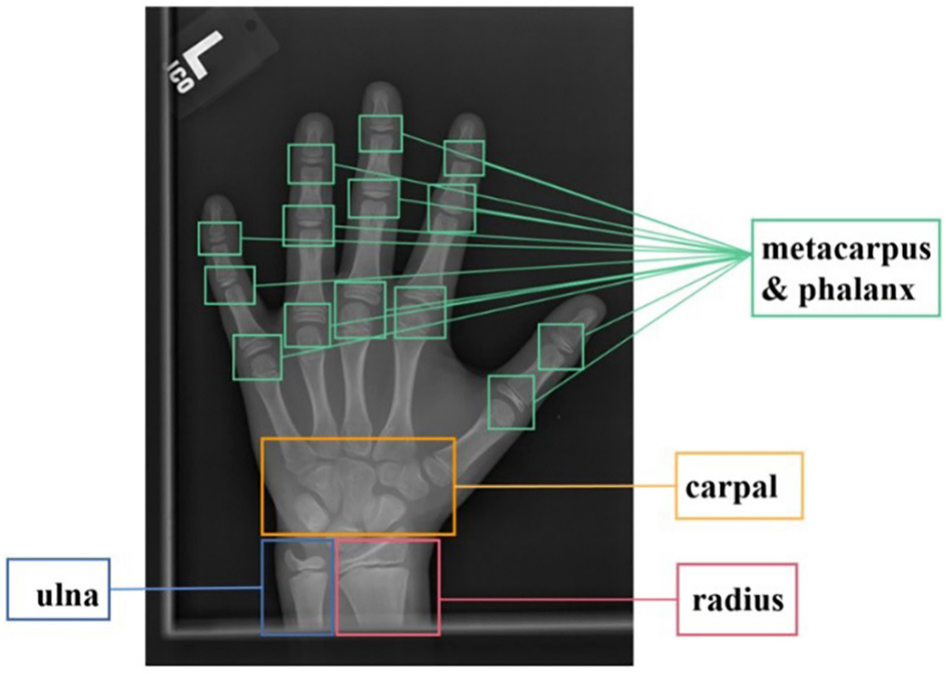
ROIs of bone X-ray images for bone age assessment.
